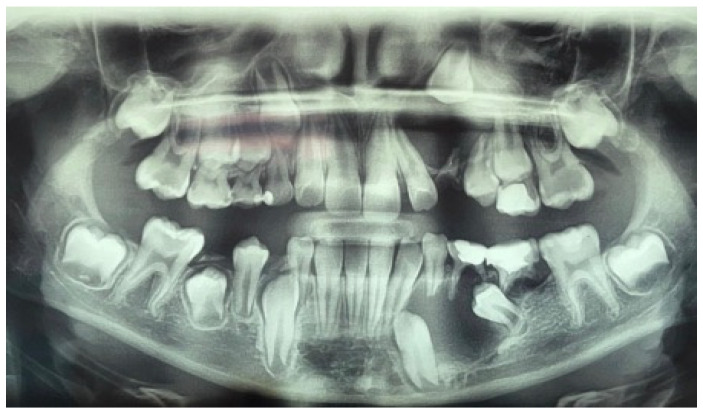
Preoperative panoramic radiograph of a ten-year-old boy showing unicystic radiolucencies on the left side of the maxilla and mandible.
